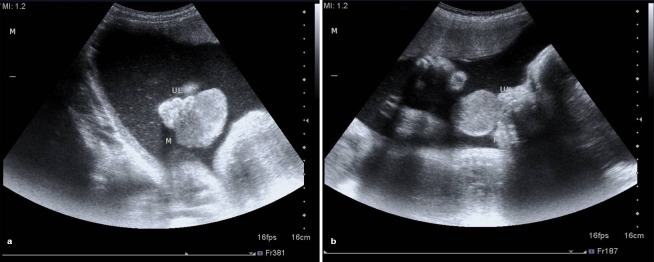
Sonography at 35 weeks of gestation, showing a well-defined, lobulated and hypoechoic mass protruding from the mouth of the fetus in axial (a) and sagittal (b) views.
